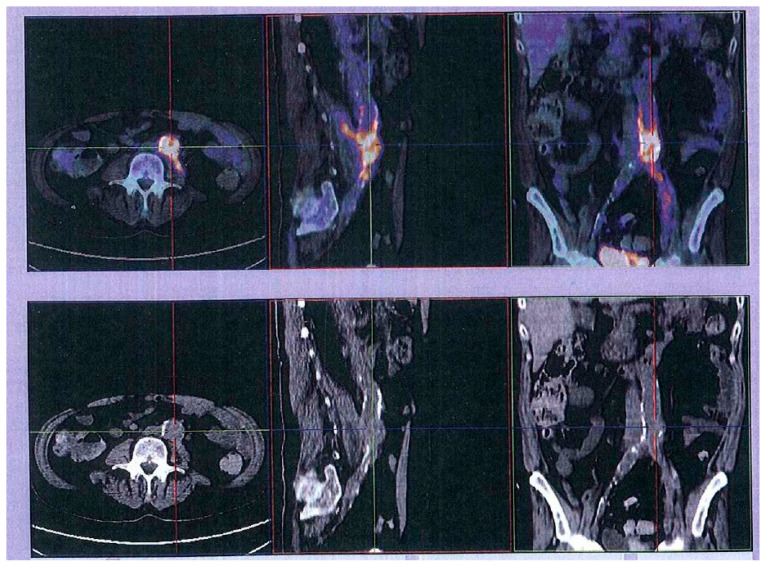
18F-FDG PET/CT revealing hypercaptation along aortic prosthesis extending into common and left external iliac arteries.
